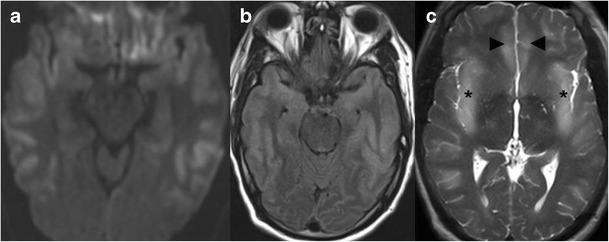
A 42-year-old male with adult onset ornithine transcarbamylase deficiency who presented with acute confusional state. Restricted diffusivity (a) and increased FLAIR signal (b) in the temporal lobes, as well as increased T2 signal in insular and cingulate gyri (c, *asterisks* and *arrowheads*, respectively). Note symmetrical distribution and prominent cortical involvement, as opposed to Fig. 3, which shows Herpes encephalitis
